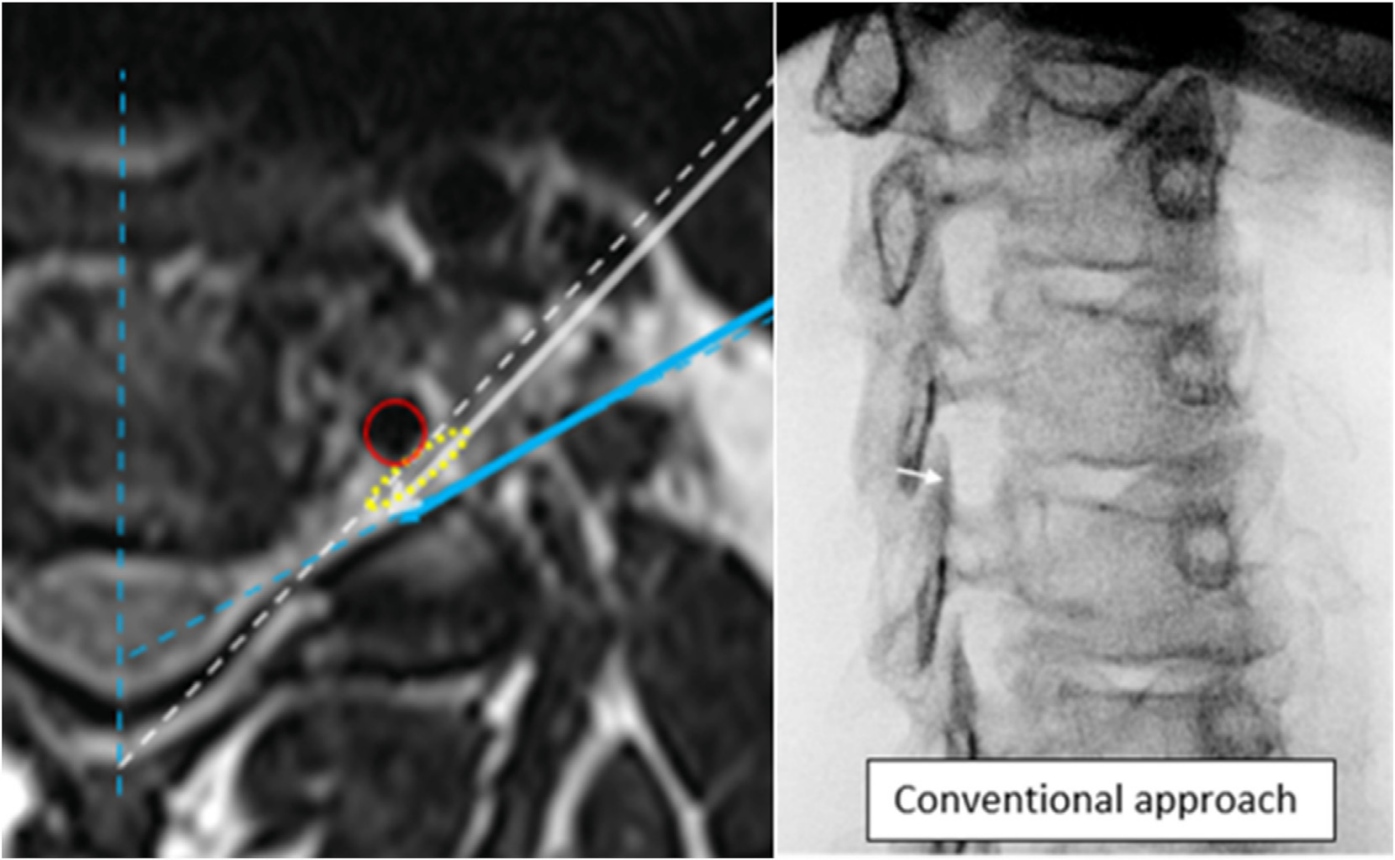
T2 axial MRI of a left C4/5 needle trajectory and corresponding conventional approach fluoroscopic oblique view demonstrating the higher risk of the conventional approach to contact the spinal nerve. MRI image demonstrates conventional approach (solid white arrow) compared to modified approach (solid blue arrow). To maximize the width of the visualized foramen on the fluoroscopic image, the oblique angle under fluoroscopy would be the same oblique angle as the lamina (dashed white line on MRI). Note that even with the conventional needle target point of one to two needle widths posterior to the visualized ventral portion of the SAP as indicated by the fluoroscopic image (arrow target point), the corresponding needle trajectory on the axial MRI contacts the neural tissue (yellow dotted oval). This contrasts with the modified approach needle trajectory (solid blue arrow) which remains clearly posterior to the neural structure. (For interpretation of the references to colour in this figure legend, the reader is referred to the Web version of this article.)
